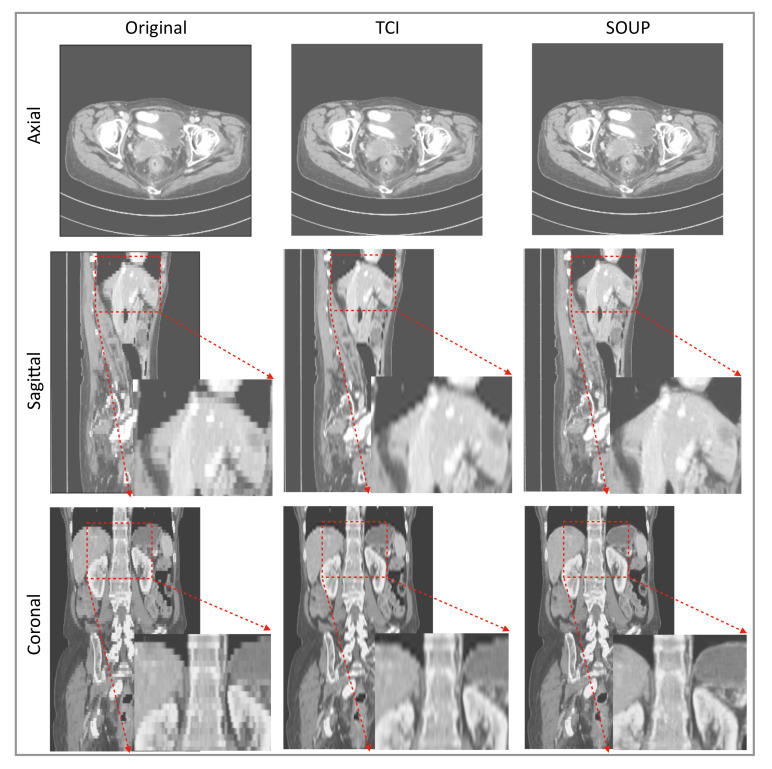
Example of SR interpolation on CT with a sampling factor equal to 4.8. Edges and textual details are better resolved by SR. Blurring is reduced. TCI: tricubic interpolation.
